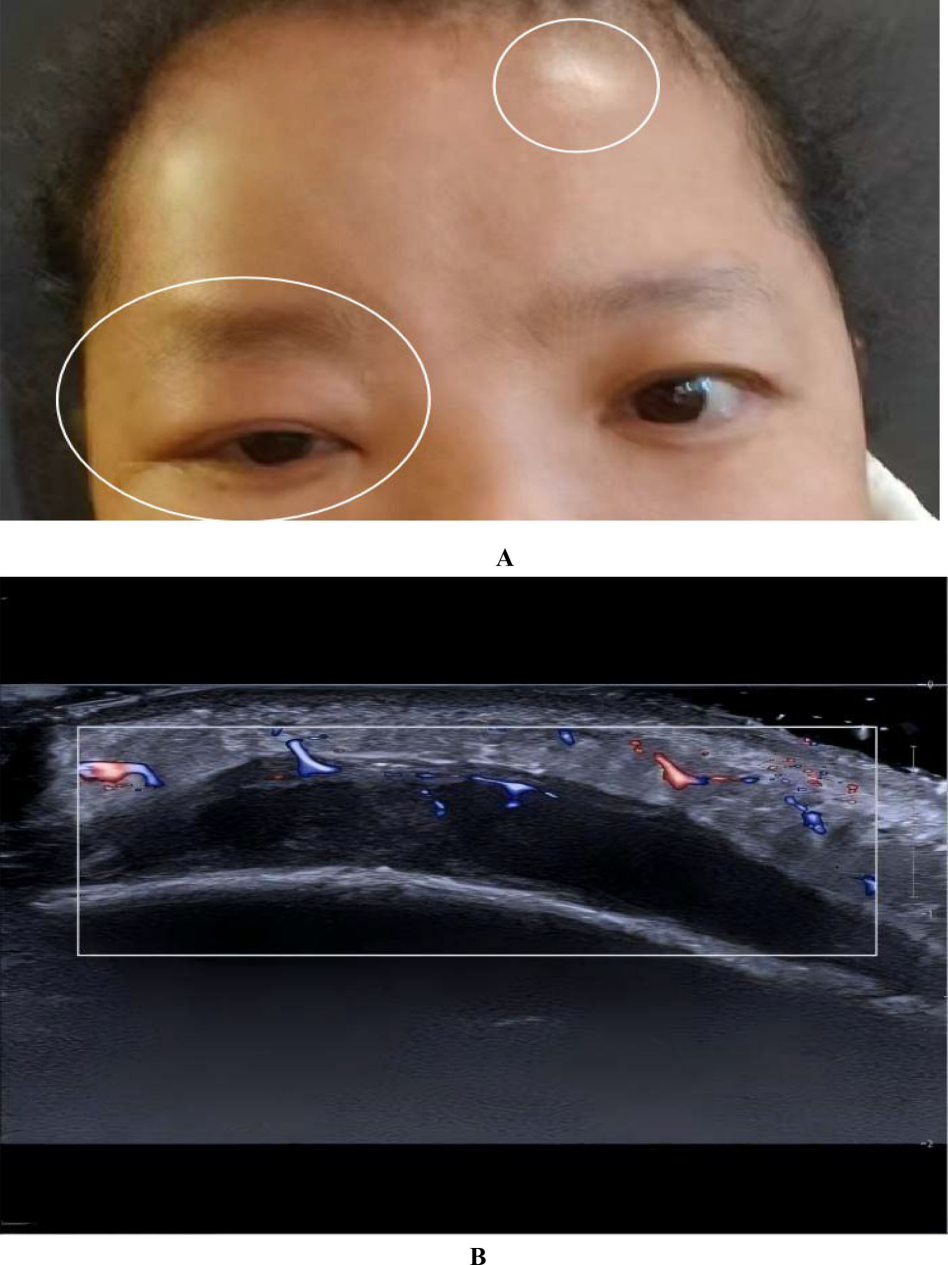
A single small nodule is present on the left frontal area, approximately 1×2 cm in size, white in color, with relatively clear borders. The surface shows no obvious ulceration or purulent discharge. Simultaneously, redness and swelling appeared around the right eyelid (A). The superficial tissue ultrasound suggested thickening of the subcutaneous layer in the frontal area, with a maximum thickness of approximately 4.9 mm. The echogenicity was reduced, and punctate or linear blood flow signals were observed within (B).
